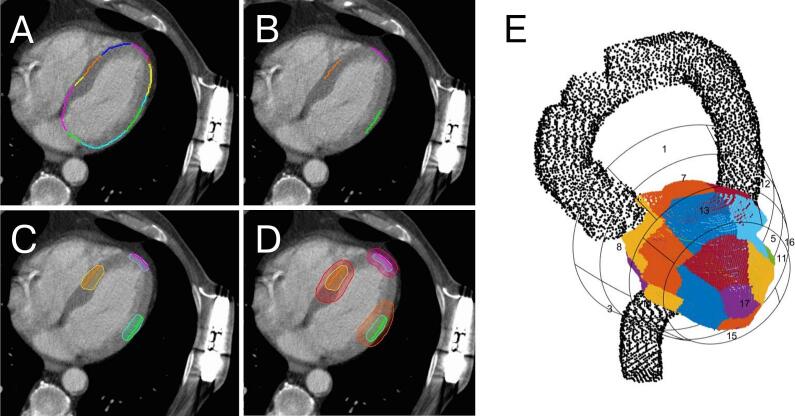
Creation of hypothetical targets in three representative heart segments. A) Semi-automated transfer of all 17 segments (American Heart Association) of the left ventricle with CARDIO-RT to the cardiac CT (transversal slice shown, segments in assorted colors; S-ICD left axilla). B) The representative segments 8 (septum, orange), 11 (left lateral, green), and 17 (apical, magenta). C) Transmural expansion to CTVs of the three representative segments. D) Final PTV (CTV + 5 mm). E) Volume rendering of the left ventricle and the semi-automatically transferred 17 segments (CARDIO-RT). Black: aorta. (For interpretation of the references to colour in this figure legend, the reader is referred to the web version of this article.)
